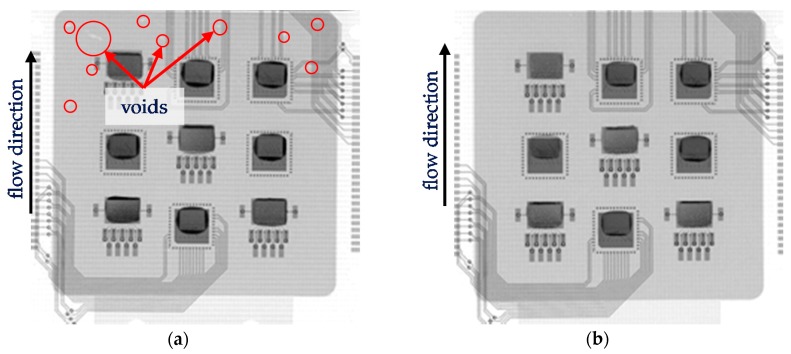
X-ray image from a PCB panel encapsulated using (a) 10 bar and (b) 30 bar cure pressure. Red circles marks voids.
